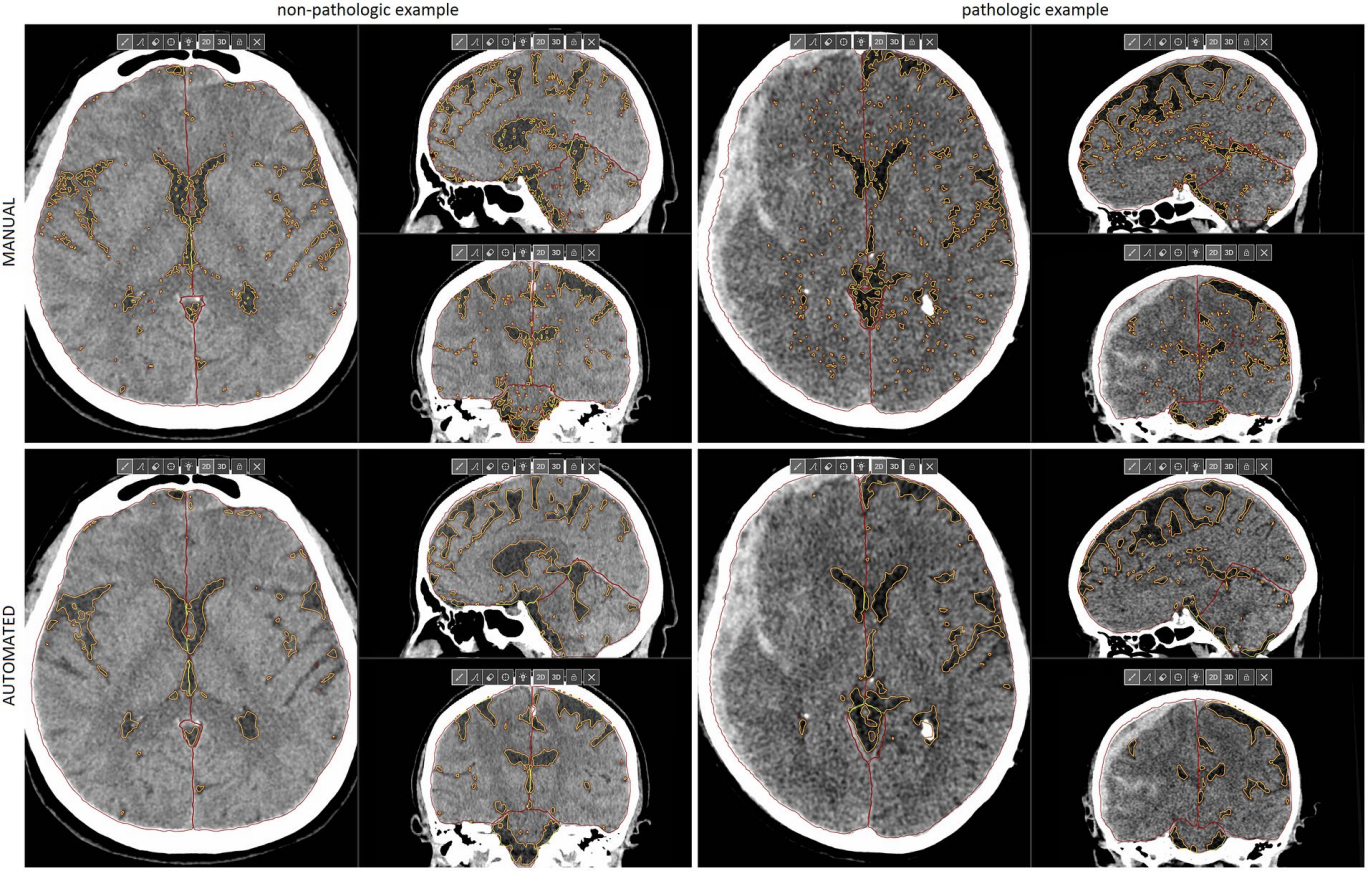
Multiplanar reconstructions of manual (upper) and automated (lower) segmentations of IC compartments and CSF in the non-pathologic (left) and pathologic example (right) of emergency CT scans. The latter example shows a right-sided acute SDH with significant mass effect that requires neurosurgical treatment, despite a relatively low midline shift. Reduced IC reserve in the right supratentorial compartment is well visualized. SDH, subdural hematoma, CSF, cerebrospinal fluid, CT, computed tomography, IC, intracranial. This figure is original to this submission so no credit or license is needed.
